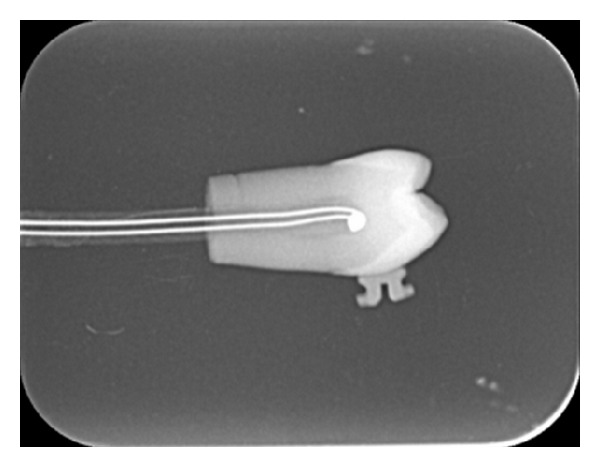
Radiographic image of the thermocouple positioned inside the pulp.
